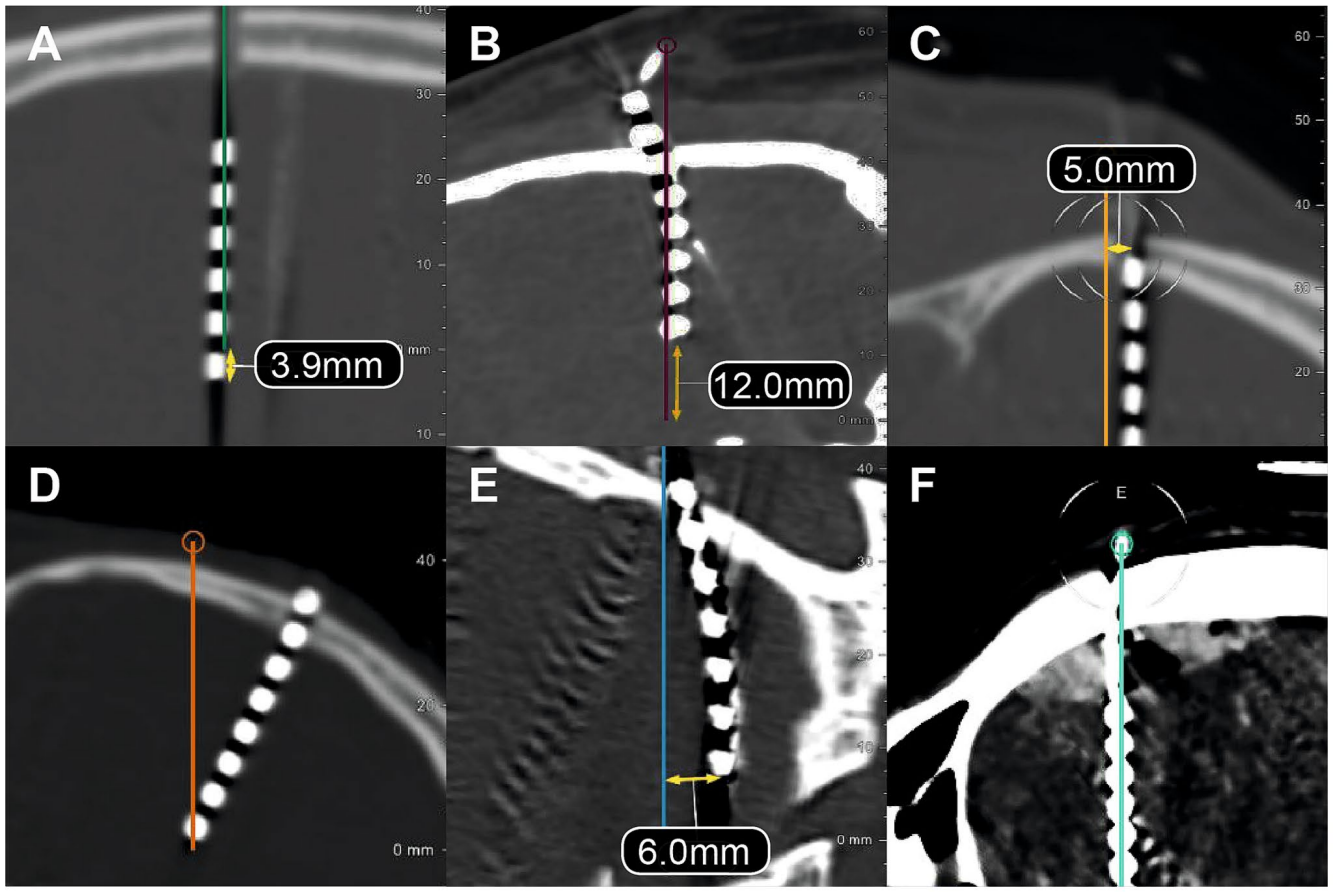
Different electrode placement error types. Postoperative CT scans fused with preoperative trajectory planning show various types of electrode placement errors. (A) Electrode placed too long, extending beyond the planned target. (B) Electrode placed too short, failing to reach the planned depth. (C) Entry point deviation resulting in an electrode positioned parallel to the planned trajectory due to an error in the X or Y coordinate. (D) Entry point deviation where the electrode still reaches the target due to a ring or arc angle error. (E) Deviation from the intended target due to trajectory misalignment. (F) Epidural hematoma observed postoperatively as a complication of implantation.
